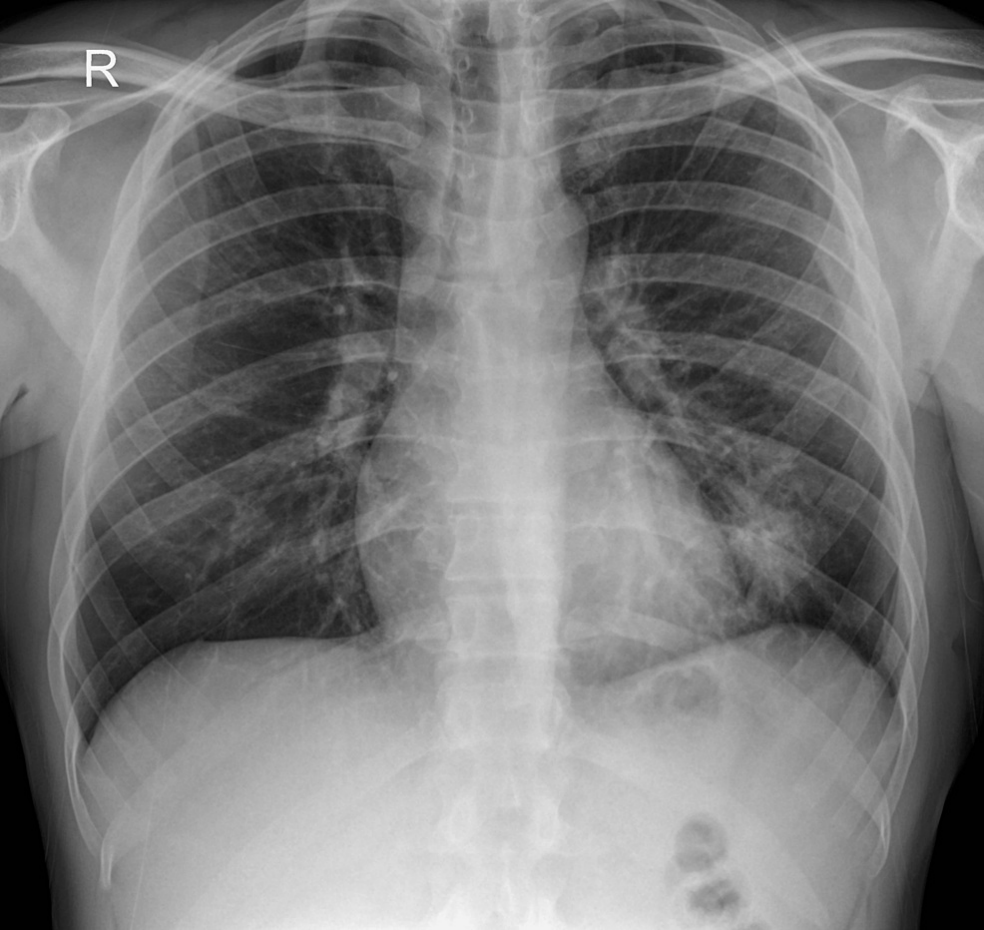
Chest X-ray after the hospital stay After two years, there seems to be no major sequel on thoracic imaging.
